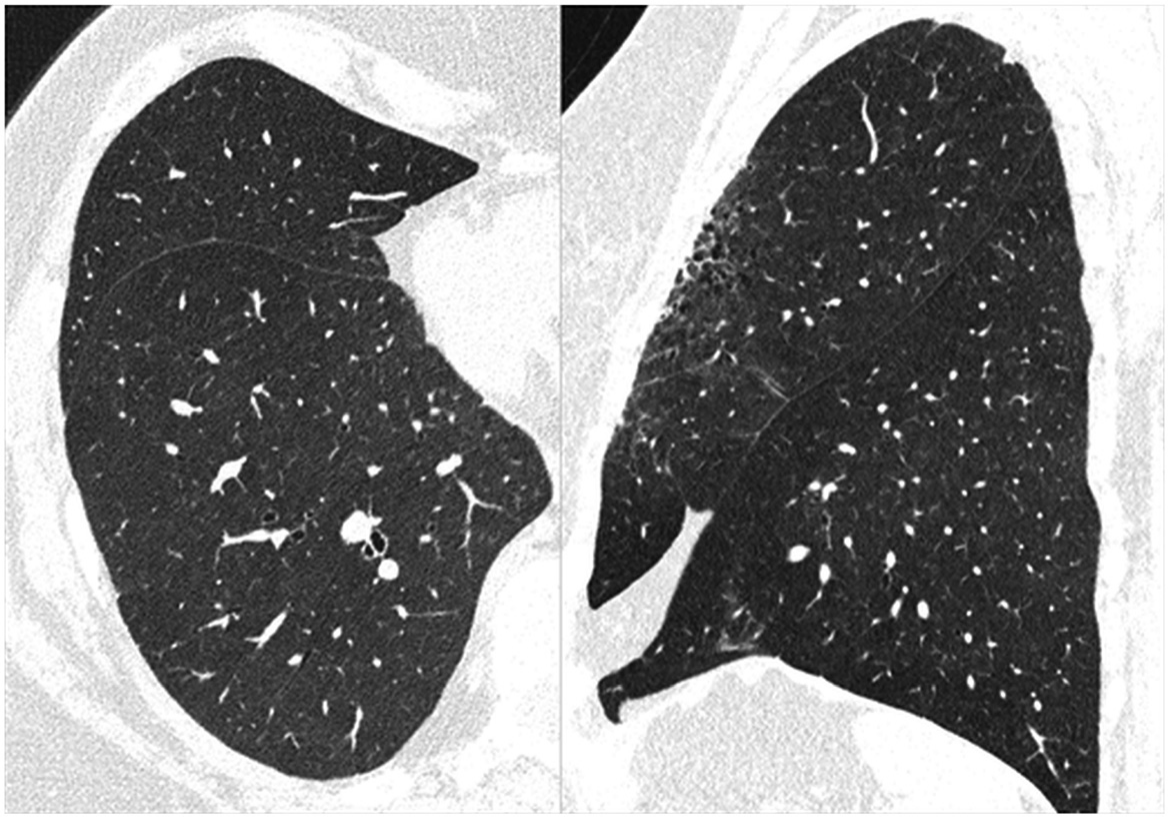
Lung images of a 74-year-old woman with breast cancer and signs of fibrosis after radiation therapy, acquired with a single-source CT prototype with photon-counting detector. Data acquisition: 120×0.2  mm collimation, 0.3 s rotation time, ${\mathrm{CTDI}}_{\mathrm{vol}}=3.89\;\mathrm{mGy}$, DLP=126  mGycm. Image reconstruction: sharp convolution kernel, 1024×1024 image matrix, 0.4 mm slice width. Excellent visualization of fibrosis and fine details such as fissures is achieved. Images courtesy of Dr. J. Ferda, Pilsen University, Czech Republic.
